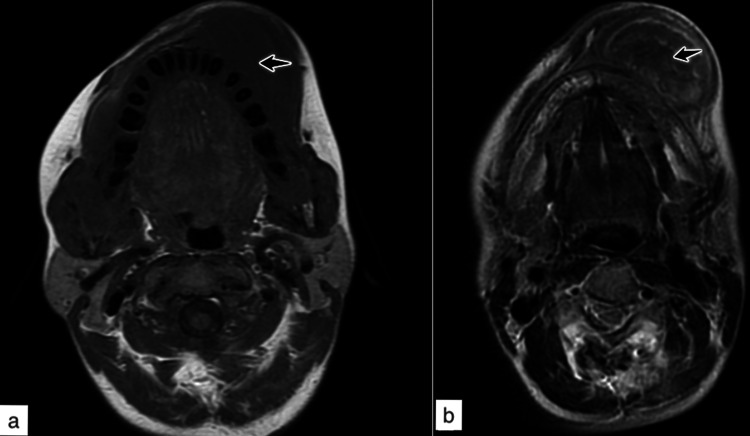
MRI face and neck (axial views) – (a) T1W and (b) T2W. Intermediate to low signal intensity multiple variable sized lesions involving maxilla and mandible.
